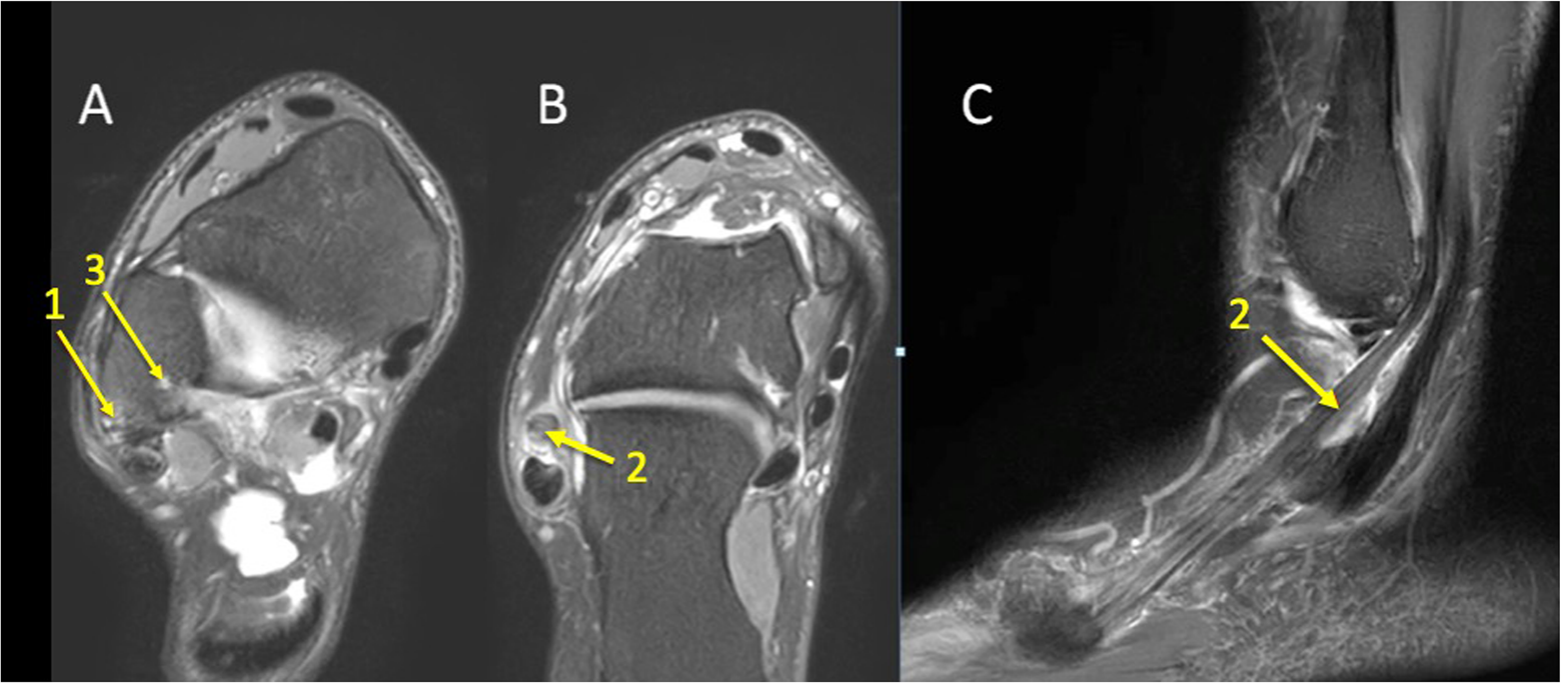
A 59-year-old female with 10-month history of lateral ankle pain and suspicion of an osteochondral lesion. MRI showed tendinopathy of the peroneus brevis tendon. (1) BME in the fibula adjacent to (2) the tendinopathy of the peroneus brevis tendon. (3) A traction cyst at the attachment of the posterior talofibular ligament
